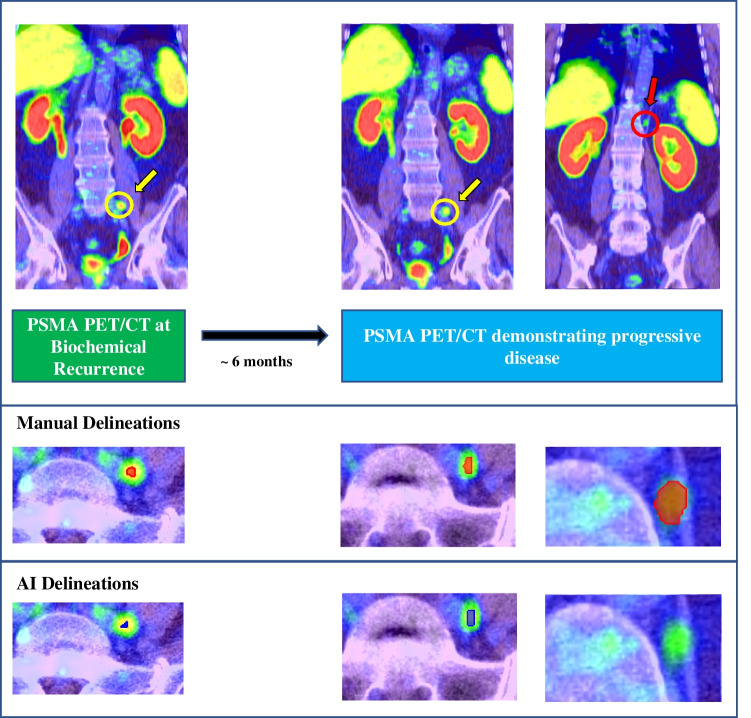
Case example of a patient demonstrating RECIP-PD according to manual interpretation, but not according to the AI model. The top row shows coronal slices of identified disease sites, by manual interpretation, at baseline and follow-up imaging. Manual and AI delineations of those disease sites in axial slices are provided directly underneath in red and dark blue, respectively. This patient (male, 68 years old, Gleason score = 9, PSA at referral = 0.23 ng/mL) presented with a single lesion in the left iliac node (yellow circle and arrow, SUV_max_ = 14.1) at baseline imaging which was successfully detected by the AI model. This lesion was also identified on PSMA PET/CT imaging 6 months later (yellow circle and arrow, SUV_max_ = 9.9) by both manual and AI scan interpretation; however, the patient also developed a new nodal disease site above the diaphragm which was only identified by human interpretation and not by the AI model (red circle and arrow). This false negative by the AI model, perhaps caused by the overall lower uptake of this lesion (SUV_max_ = 2.8), led to the discordance in RECIP classifications for this patient (RECIP-PD for manual, non RECIP-PD for AI). Despite the discordance in RECIP classification, there was concordance on whether the patient had a ΔTTV ≥ 20% between initial and follow-up imaging (ΔTTV_man_ = 325%, ΔTTV_AI_ = 54%)
